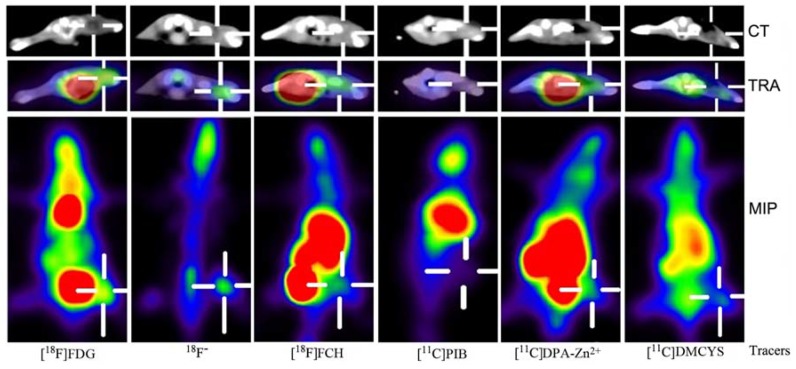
The transverse (TRA) CT and PET/CT fusion images, and the coronal maximum intensity projection (MIP) images in IM (cross-shaped lines) with multiple tracers ([^18^F]FDG, ^18^F^−^, [^18^F]FCH, [^11^C]PIB, [^11^C]DPA-Zn^2+^ and [^11^C]DMCYS) at 60 min post-injection. The high uptake of ^18^F^−^ and [^18^F]FDG was observed. In addition, the moderate uptake in [^18^F]FCH, [^11^C]DPA-Zn^2+^ and [^11^C]DMCYS, and almost no uptake of [^11^C]PIB in IM were demonstrated. Moreover, the intense uptake of [^18^F]FCH and [^11^C]DPA-Zn^2+^ in abdomen was found. The background activity of ^18^F^−^ was extremely low.
